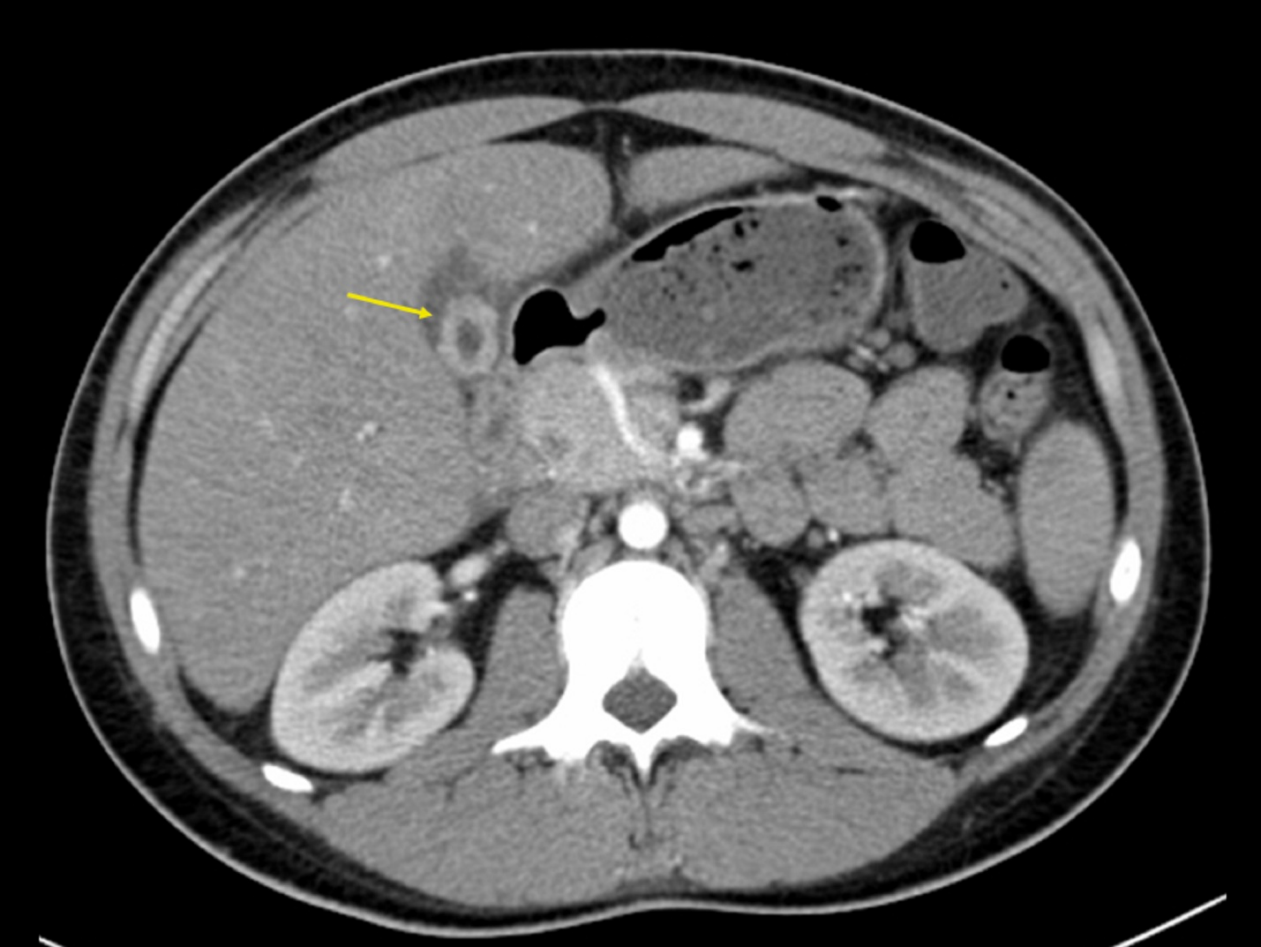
A Computed Tomography Scan of the Abdomen The arrow demarcates a thick-walled gallbladder with a rim of pericholecystic fluid
